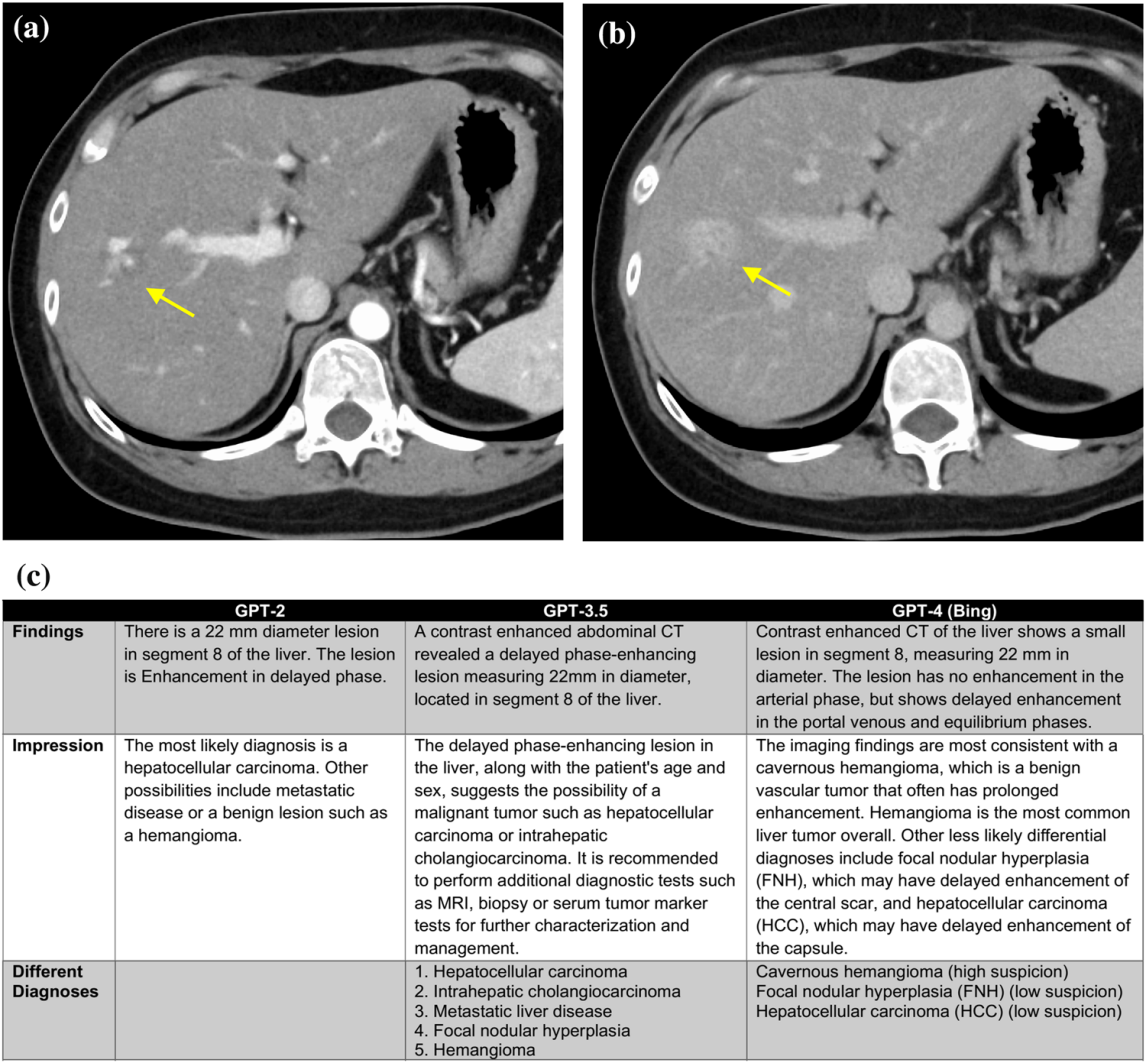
A 31-year-old female with a hepatic hemangioma. The contrast-enhanced CT arterial phase (a) shows heterogeneous enhancement within the lesion, and the venous phase (b) reveals a generally stronger enhancement than the liver parenchyma, consistent with typical findings of a hemangioma. Generated radiology reports by GPT series (c) are also shown. The information inputted besides the prompt is “Age (years): 31; sex: female; modality: contrast enhanced CT; location: segment 8 in the liver; diameter: 22 mm; findings: delayed phase-enhancing lesion”. In the GPT-2 generated report, a list of differential diagnoses is not even created, and the impression primarily suspects hepatocellular carcinoma. In the GPT-3.5 generated report, although the format is well organized, hepatocellular carcinoma is still listed as the top differential diagnosis. The GPT-4.0 generated report is generally quite good, with reasonable differential diagnoses
